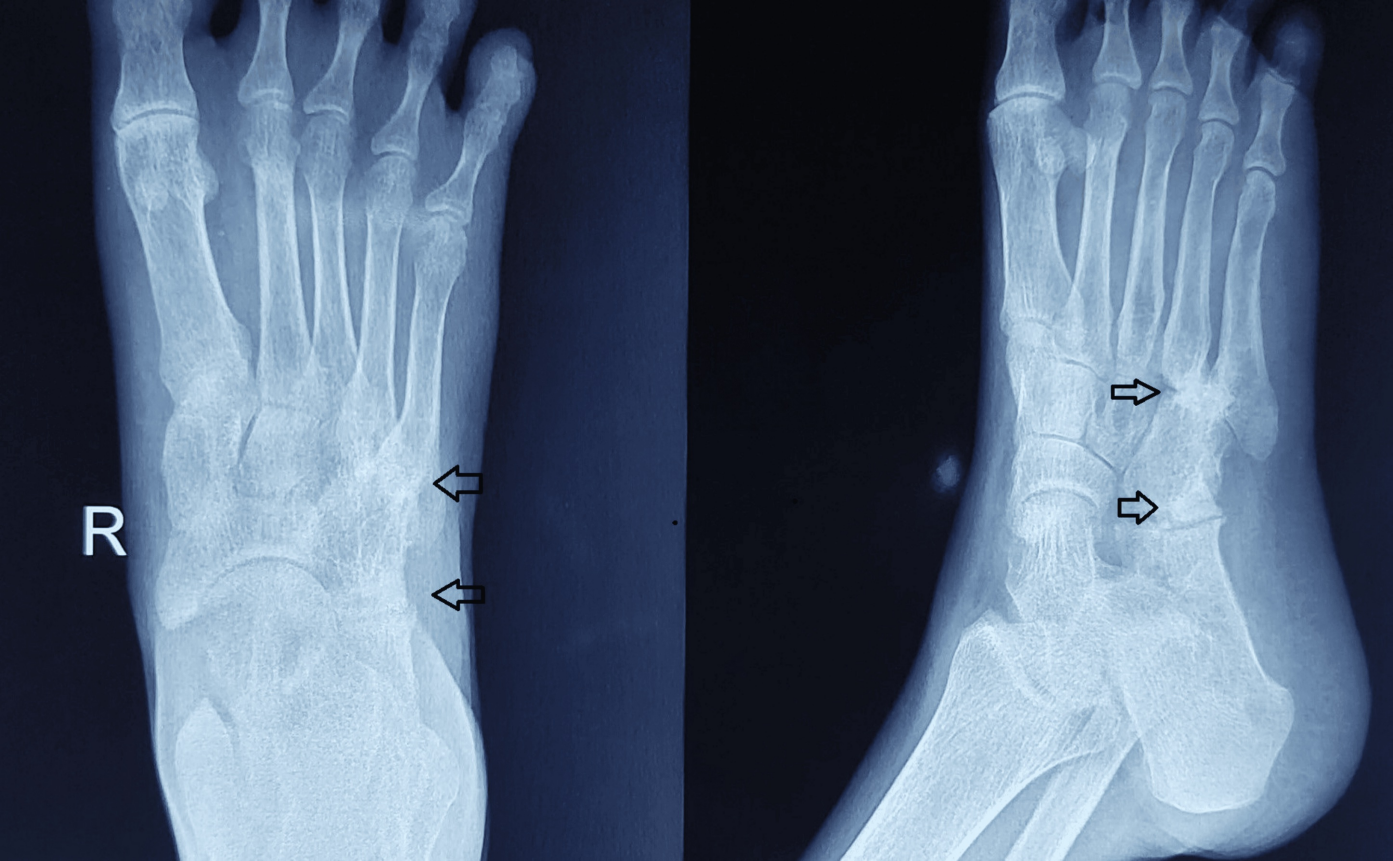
Postoperative follow-up X-rays at the 9th month show bone graft consolidation at the calcaneocuboid and tarsometatarsal joints.
